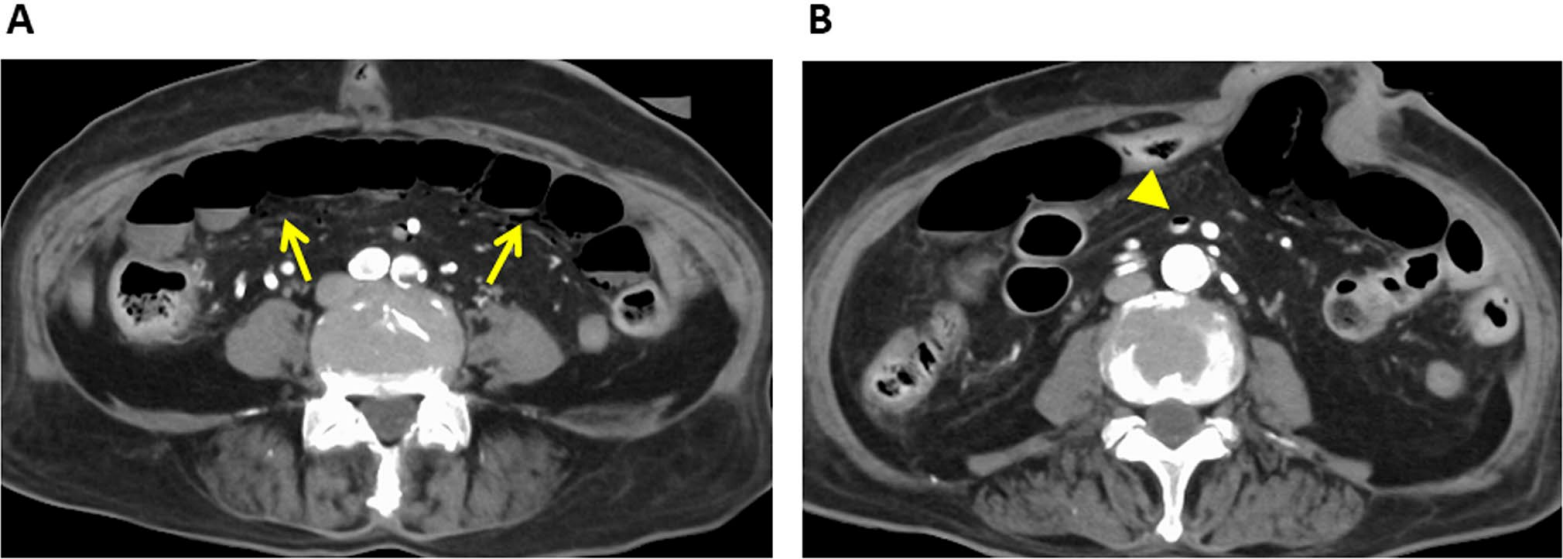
Non-occlusive mesenteric ischemia in an 87-year-old woman on dialysis for chronic renal failure. A Axial contrast-enhanced CT image shows thinning of the bowel wall (arrows) resulting in its paper-thin appearance. B Gas is seen in the superior mesenteric vein (arrow head)
